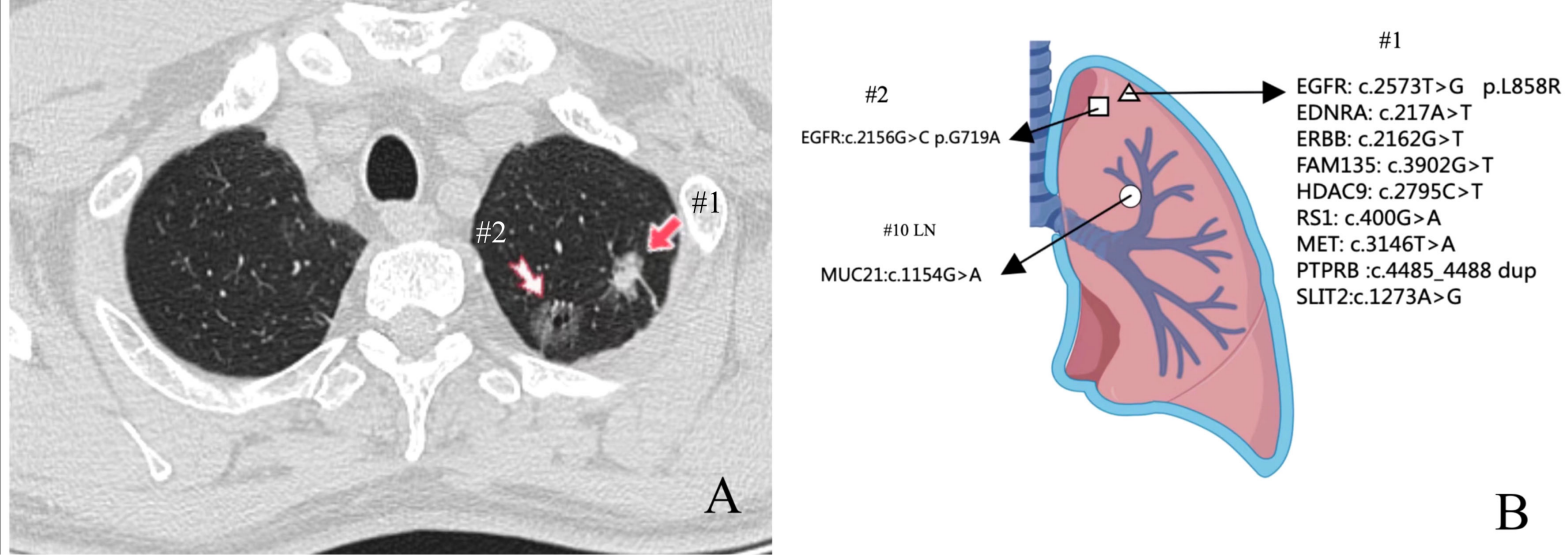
Location and next-generation sequencing (NGS) results of the three pulmonary lesions. (A) Chest computed tomography showed two ground-glass density nodules in S1 of the upper lobe of the right lung. (B) Genomic alterations detected via NGS analysis in three lesions.
